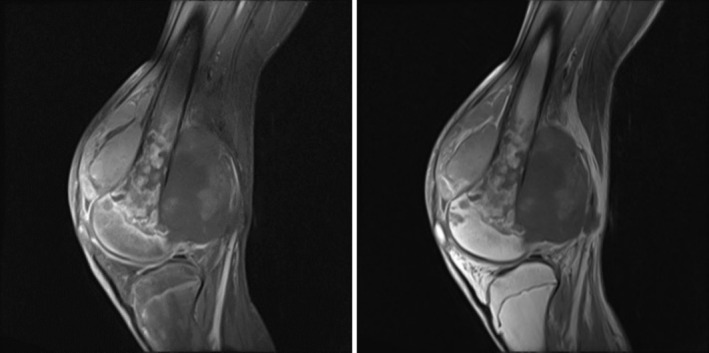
MRI of the knee showing a tumor process of the inferior femoral metaphysis enhanced after injection of gadolinium with foci of necrosis and extension to the soft parts.
